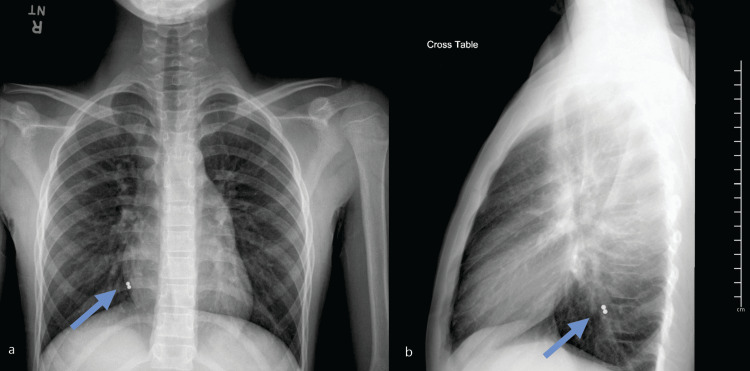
(a) X-ray AP chest: Two small circular radio-opacities (arrows), most likely ingested magnets, are seen in the right lower lobe. Hyperinflation of the lungs is noted, without consolidation. (b) X-ray lateral chest view of the circular radio-opacities. Image credit: Dr. Lena Naffaa
